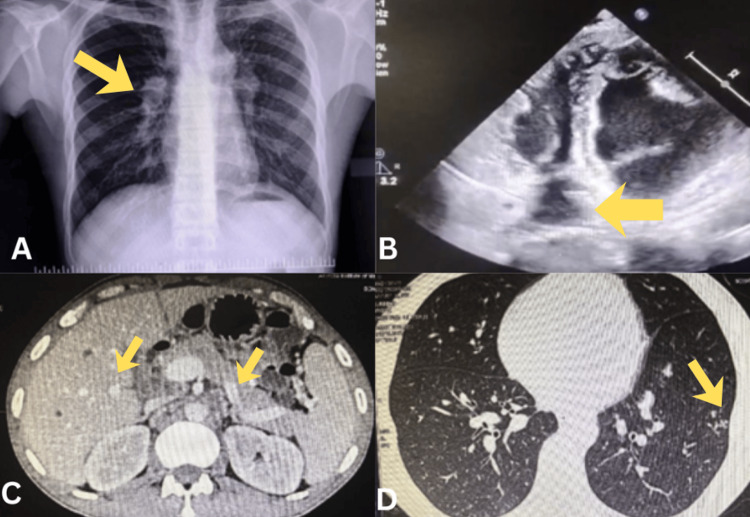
(A) Chest X-ray showing bilateral hilar lymphadenopathy (arrows indicate enlarged bilateral hilar nodes, right greater than left). (B) Transthoracic 2D echocardiogram showing a mobile vegetation (arrow) attached to the tricuspid valve leaflets, consistent with infective endocarditis; vegetation measured approximately 1.2 × 0.8 cm. (C) Contrast-enhanced computed tomography (CECT) abdomen showing conglomerate heterogeneously enhancing necrotic lymph nodes in the periportal, pre-aortic, and paraaortic locations (arrows), with multiple sub-centimetric non-enhancing hypodense lesions in the liver parenchyma consistent with hepatic granulomas. (D) CECT thorax showing centrilobular nodules with a tree-in-bud appearance in the left lower lobe (arrows), consistent with endobronchial spread of tuberculosis. Mediastinal lymphadenopathy is also visible at the carinal level.
